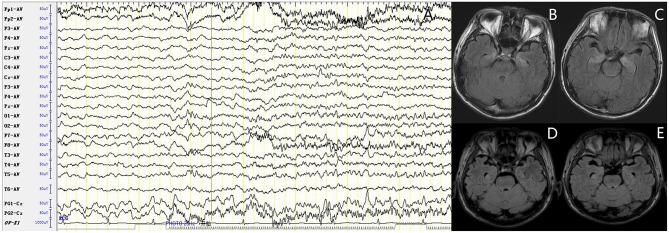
Case 4, male, 62 years old, who was diagnosed with leucine-rich glioma-inactivated 1 protein (LGI1) antibody associated autoimmune encephalitis (AE). (A) Ictal electroencephalogram (EEG) of a subclinical seizure showed rhythmic sharp wave discharges in bilateral temporal regions, with amplitude and frequency modulated gradually. (B,C) Twenty-three days after onset and before immunotherapy, brain MRI showed high T2/fluid-attenuated inversion recovery (FLAIR) signal on bilateral hippocampus. (D,E) About 3 months after onset and after immunotherapy, brain MRI showed significant improvement in the high T2/FLAIR signal on bilateral hippocampus.
